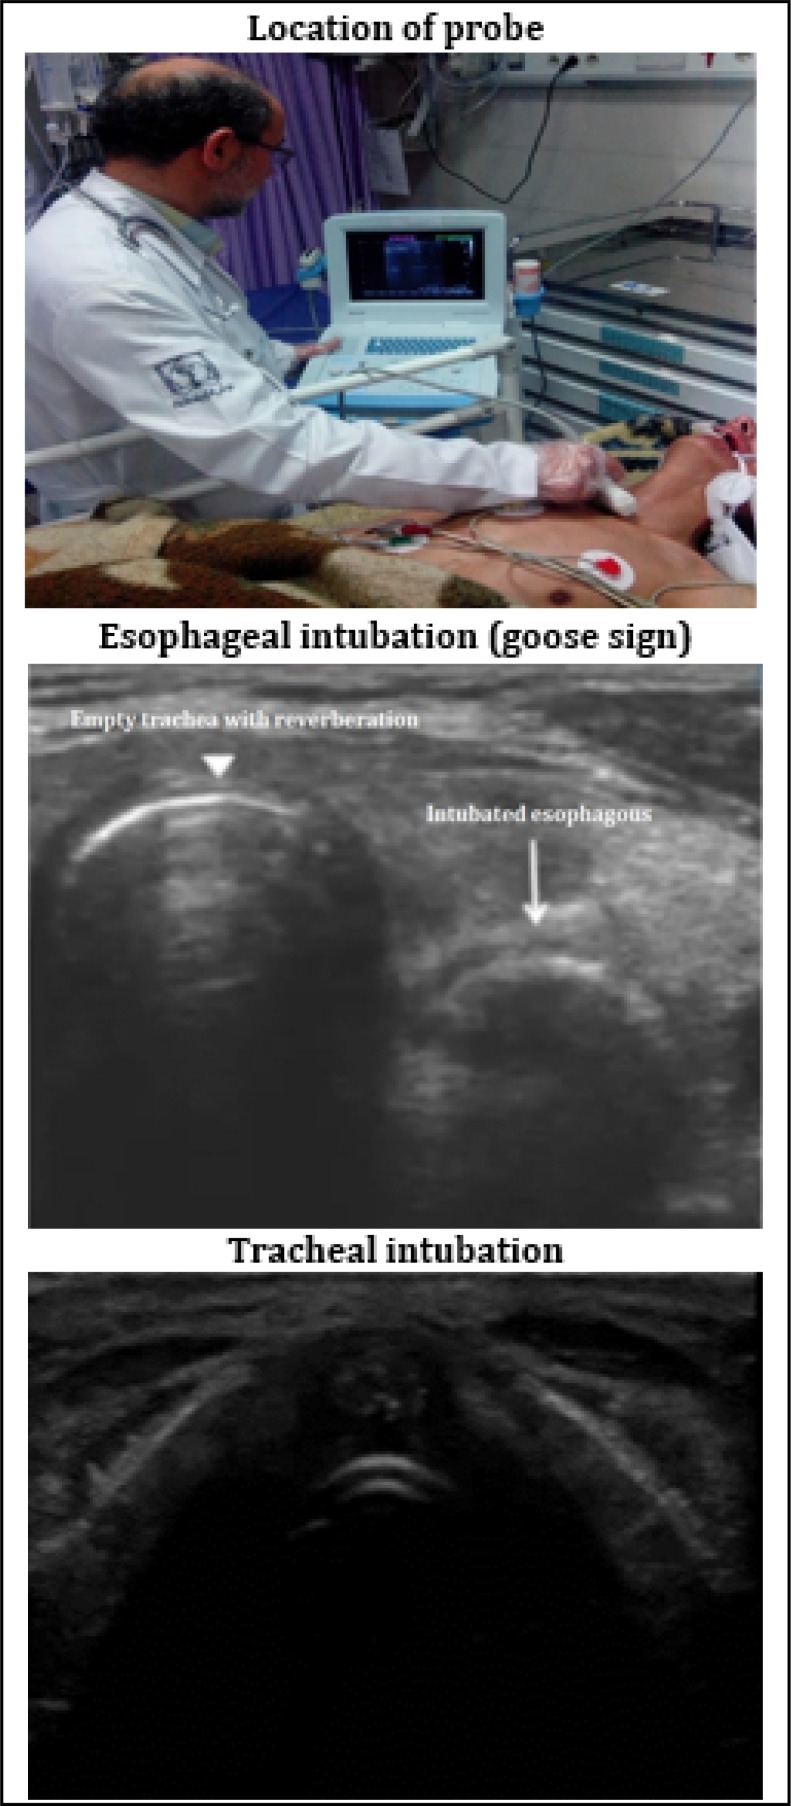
Tracheal ultrasonography.
